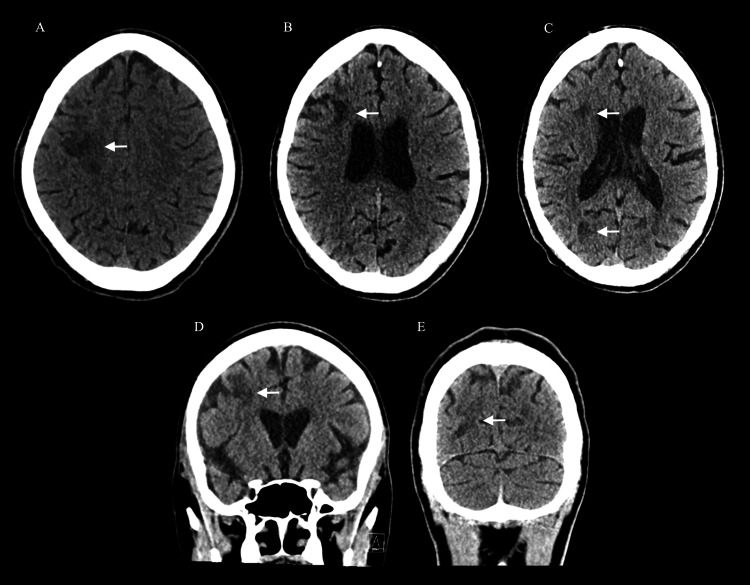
(A-C) Axial and (D, E) coronal brain CT without contrast performed on the first day demonstrating ill-defined subcortical frontal and parietal hypodensities (white arrows) Computed tomography (CT)
